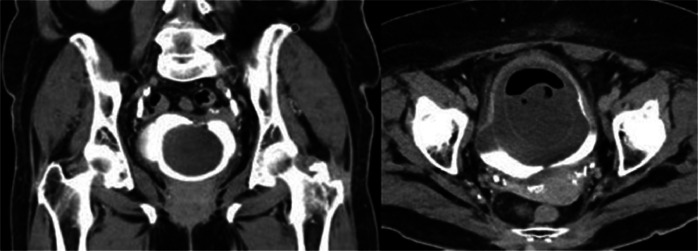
Excretory phase abdominal CT: The excretory phase revealed an endoluminal filling defect at the vesical level corresponding to the previously described bladder mass.
